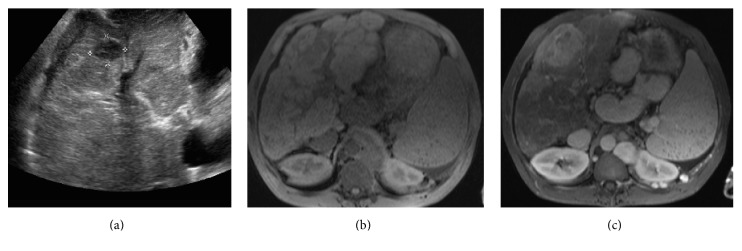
(a) Longitudinal US image of a 34-year-old male with cystic fibrosis was done as part of routine surveillance for cirrhosis in the context of stable biochemical markers. US image demonstrating a well-circumscribed hypoechoic lesion. (b and c) Noncontrast and arterial phase T1-weighted gadolinium enhanced MRI demonstrating a hyperenhancing well-circumscribed lesion in segment 4. Splenic siderotic nodules are an additional feature of portal hypertension.
